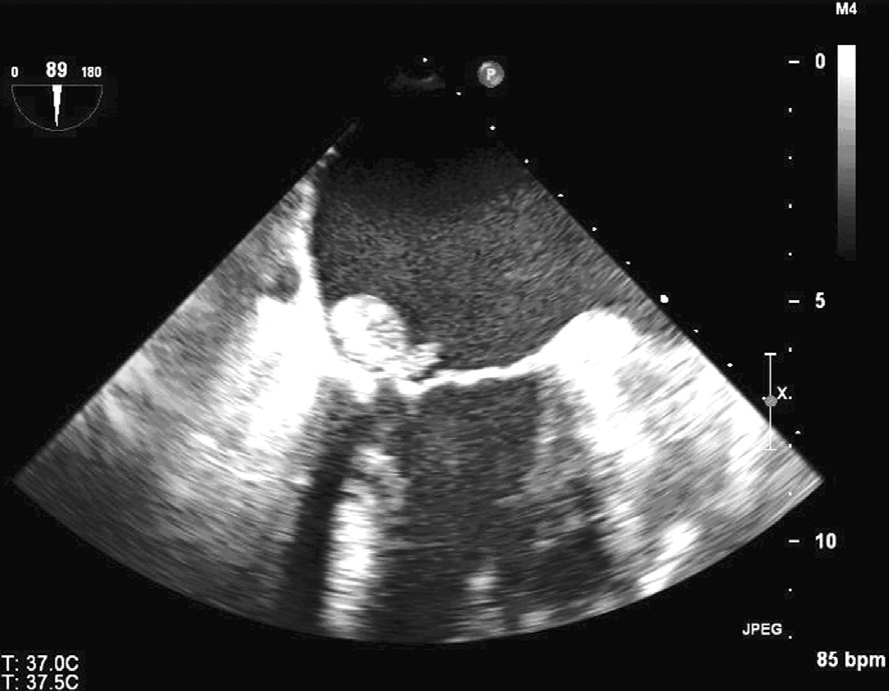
Transesophageal echocardiogram showing a 2 cm mobile mass on the atrial surface of the posterior mitral valve leaflet consistent with a vegetation.
